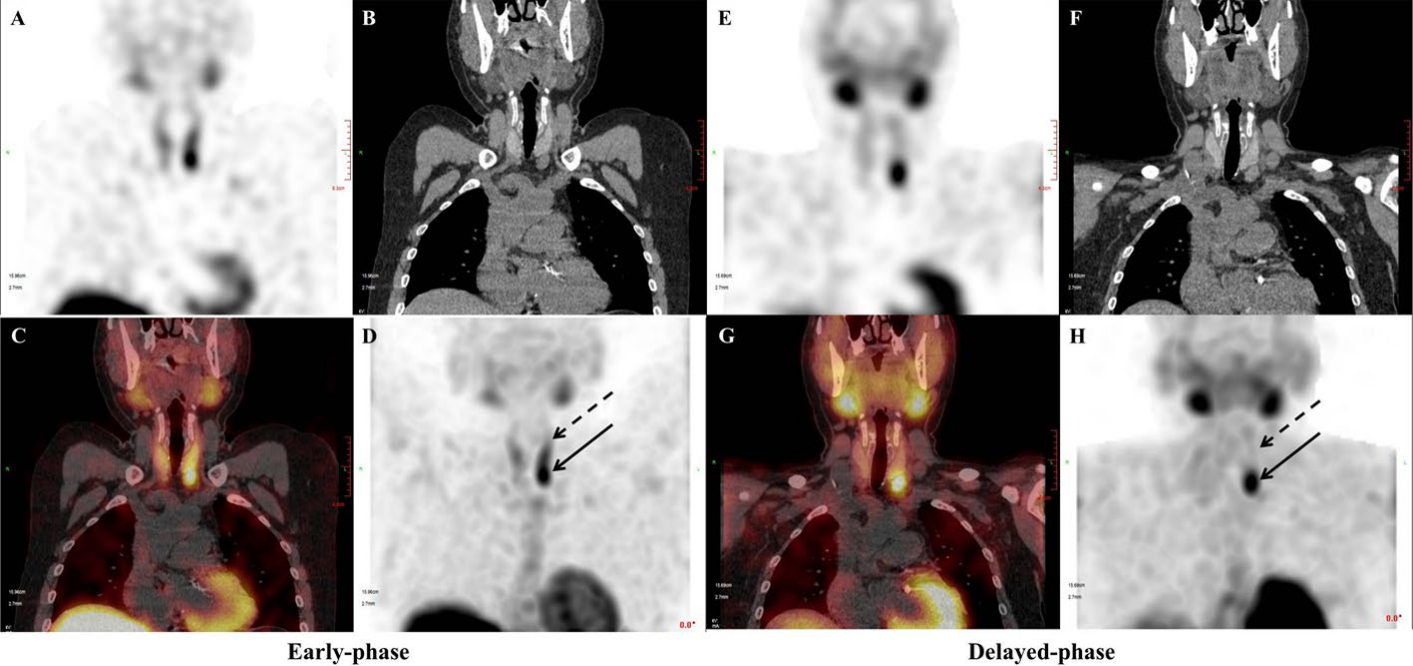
Early phase of coronal SPECT (A), coronal CT (B), coronal SPECT/CT (C) and MIP (D) images from 99mTc-MIBI displaying a clear thyroid image (dotted arrow), with focal imaging agent concentration below the inferior pole of the left lobe (arrow). Delayed phase of coronal SPECT (E), coronal CT (F), coronal SPECT/CT (G) and MIP (H) images showed significant tracer washout from the thyroid (dotted arrow) while high concentration persisted in the lesion below the inferior pole of its left lobe (arrow). *MIP* is maximum intensity projection
